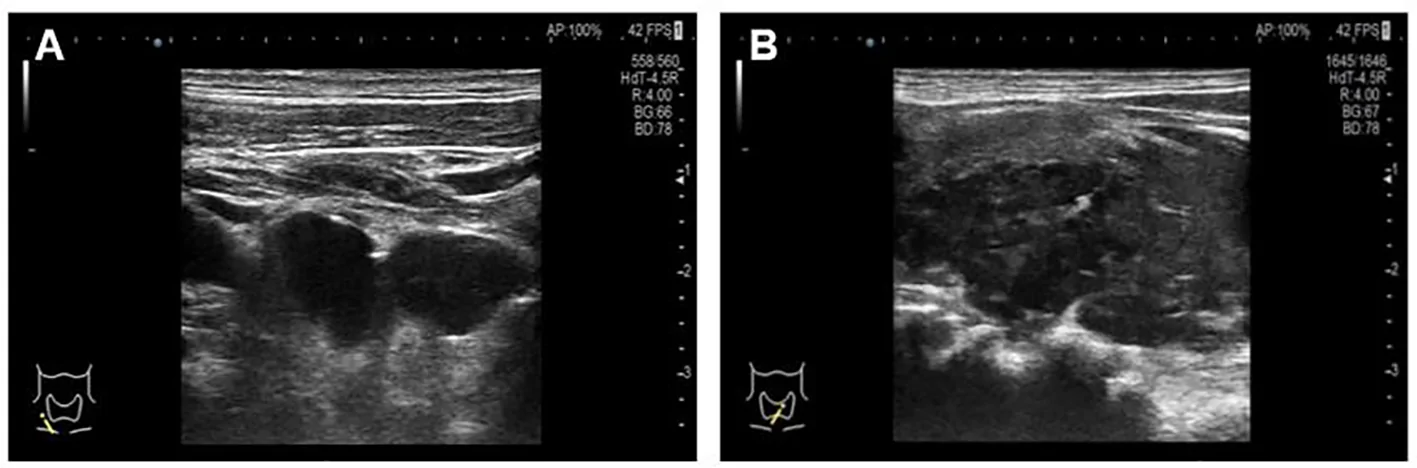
Neck ultrasound. (A) Transverse view of the left thyroid lobe: a 31 × 28 mm heterogeneous hypoechoic lesion with ill-defined borders. (B) Multiple enlarged lymph nodes are seen in level VI and pretracheal space.
